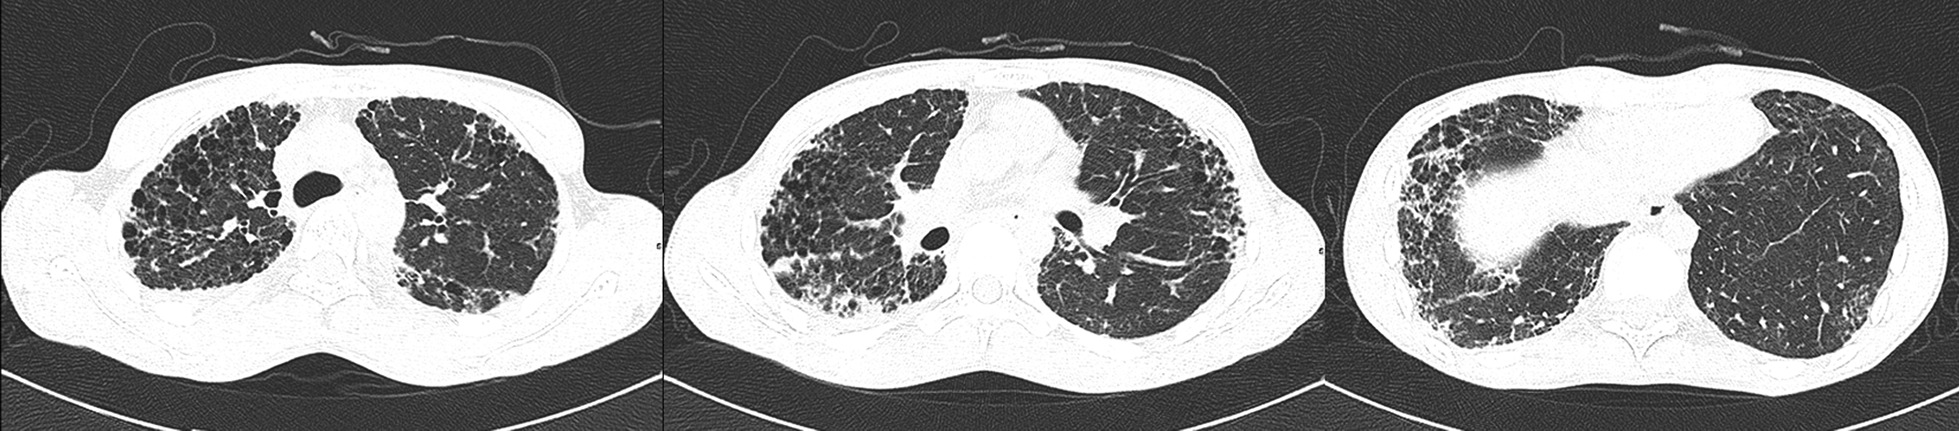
High resolution computed tomography manifestation of the patient. Chest high resolution computed tomography scan showed mid- and upper-zone predominant bilateral peripheral reticulolinear shadows, interlobular septal thickening with associated traction bronchiectasis and honeycombing, compatible with pulmonary fibrosis
